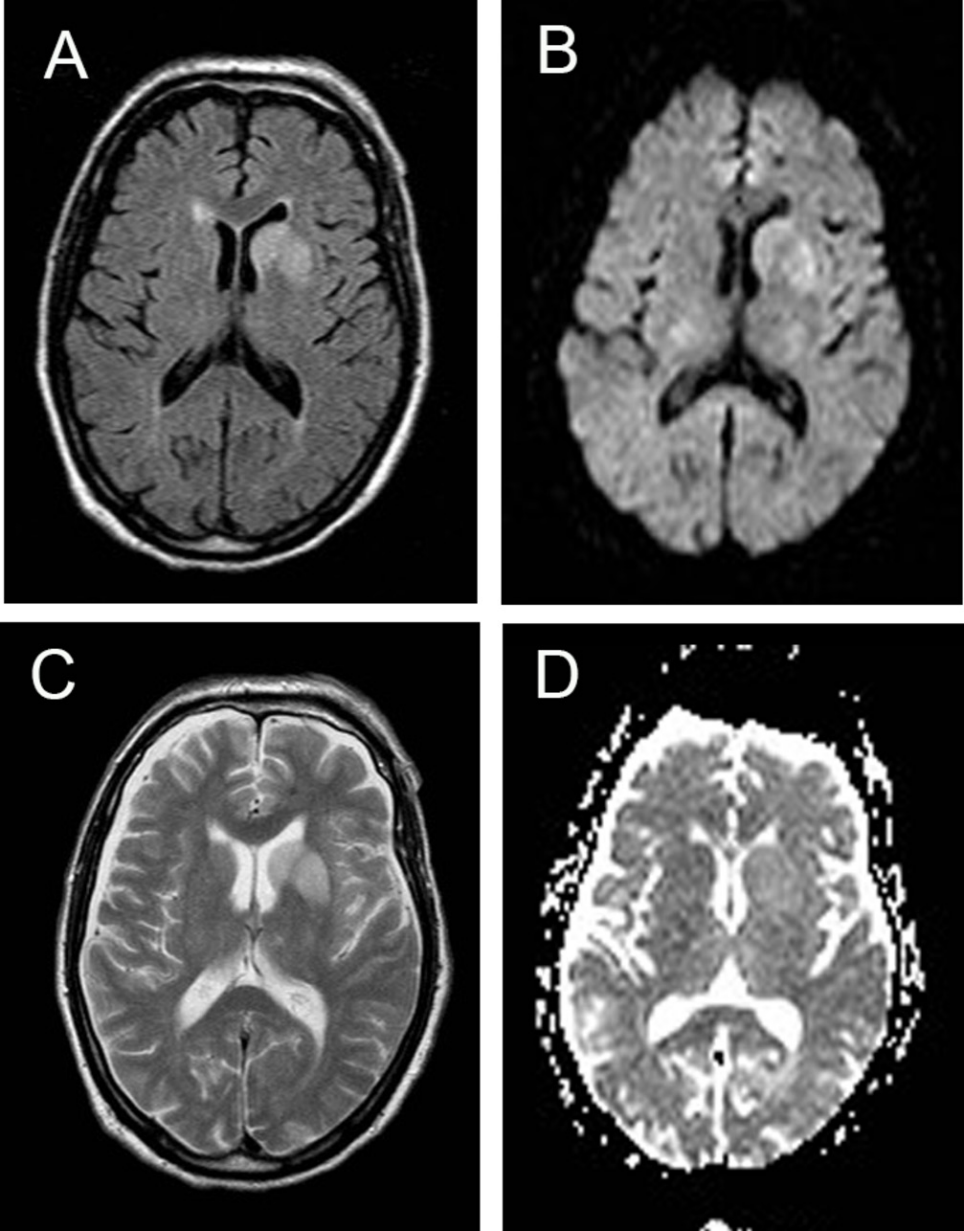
MRI in patient with autoimmune encephalopathy due to LGI1 antibodies. Note the high signal return from the swollen left basal ganglia on T2 (A), FLAIR (B) and DWI (C) sequences but restriction is not confirmed on ADC mapping (D). ADC, apparent diffusion coefficient; DWI, diffusion-weighted images; LGI1, Leucine-rich, glioma-inactivated antibodies-1.
